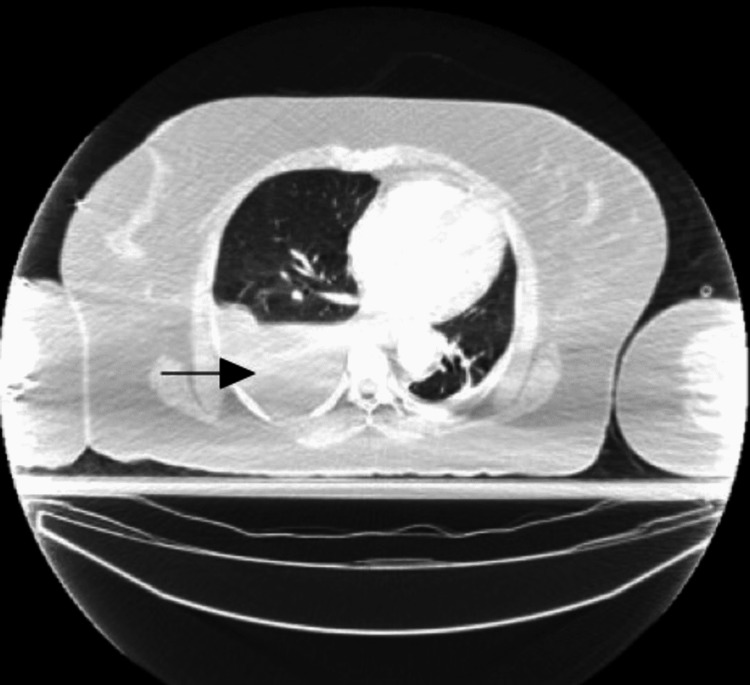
Computed tomographic angiogram of the chest with IV contrast The arrow designates a large right-sided hemothorax.
